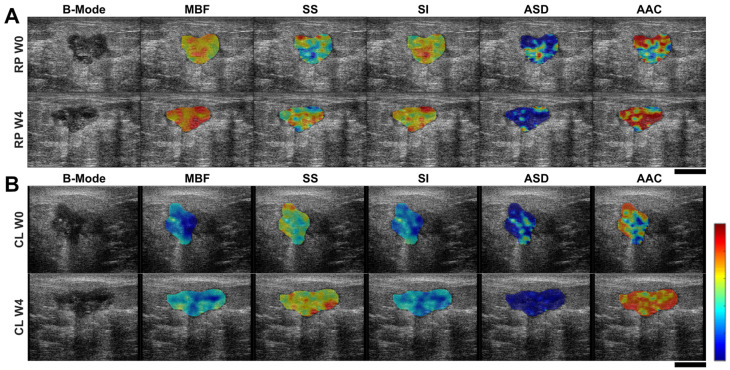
Cross-system comparison showing ultrasound B-mode images of a representative breast tumor. The tumor was imaged using both the Sonix RP (A) and the Clarius L15HD (B). Color overlays are QUS feature parametric maps (for MBF, SS, SI, ASD, and AAC) for the baseline ‘week 0’ time point (top of (A,B)) and the week 4 time point (bottom of (A,B)). The color scale shows variation in feature magnitude. The black scale bars represent 1 cm.
